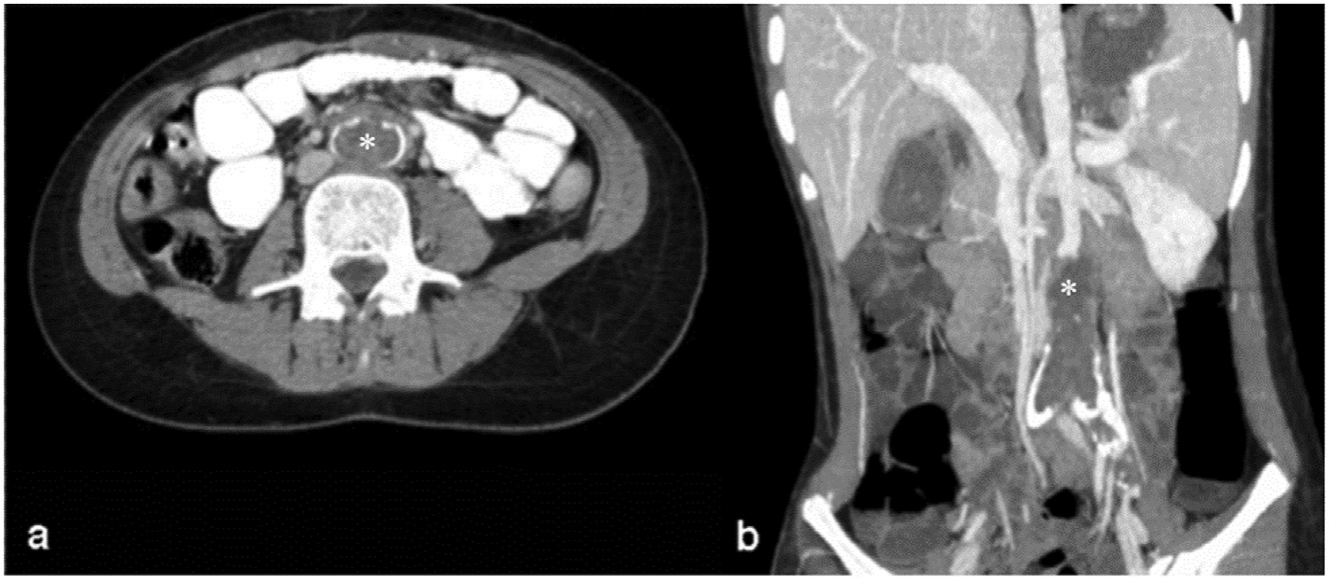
Axial (a) and coronal reformatted (b) contrast-enhanced abdominal CT scan in a 14-year-old girl shows an expansile filling defect with calcified walls in the abdominal aorta (Asterisk). The patient is a known case of generalized hydatidosis and this intra-aortic involvement has been stable for 2 years. (Photos are from our own patient(s) and they had not been published previously elsewhere.).
